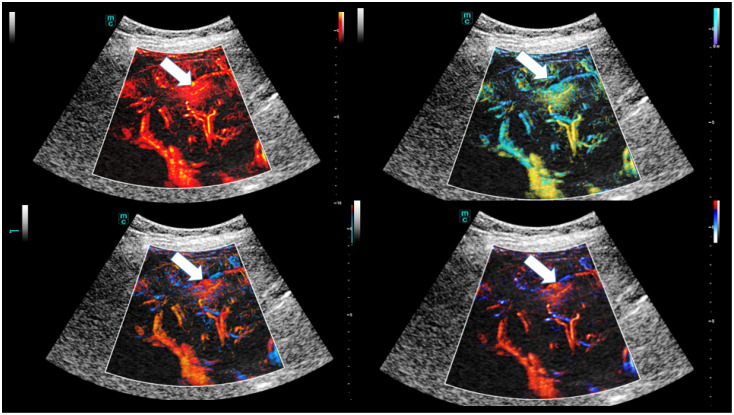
Different modalities of SR CEUS after injection of 1.4 mL ultrasound contrast agent with irregular hyperenhancement of the liver and early nodular enhancement of high-flow haemangiomas (arrows) and micro-shunts. Visualisation by dynamic SRCEUS (red colours), velocity (yellow and green), directional (blue and red), and bi-directional (blue, red, orange).
